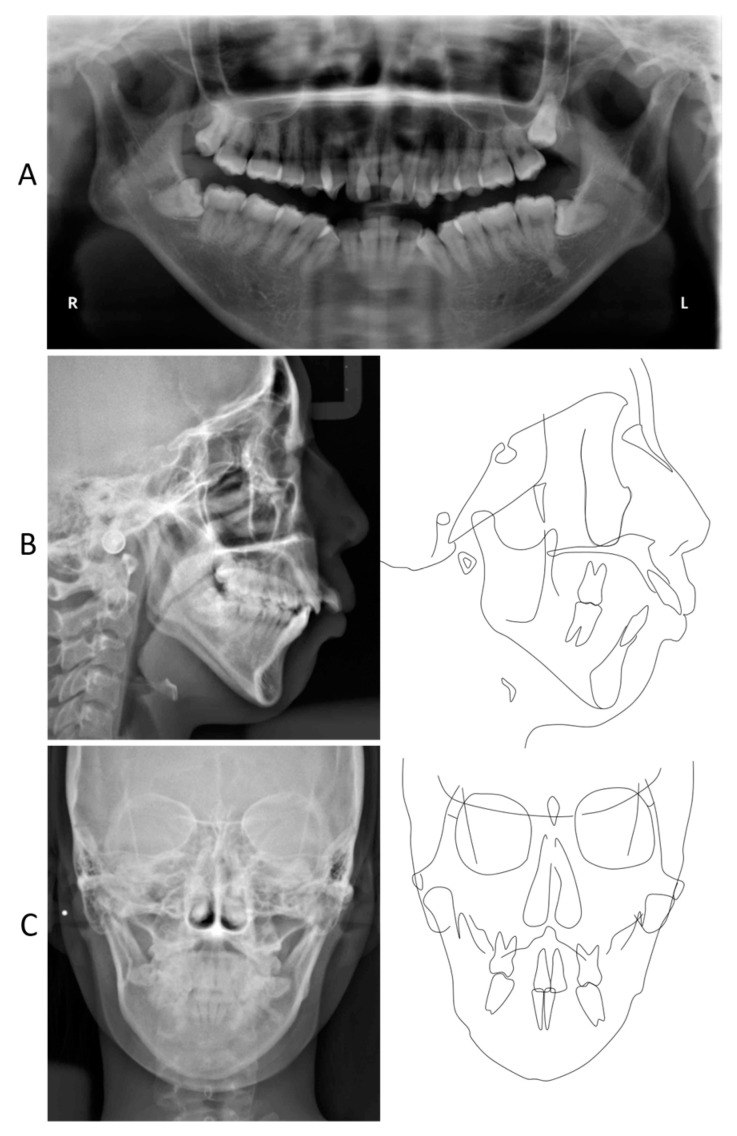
Pretreatment radiographical records. (A) Panoramic radiograph; (B) Lateral cephalogram and its tracing; (C) Frontal cephalogram and its tracing.
